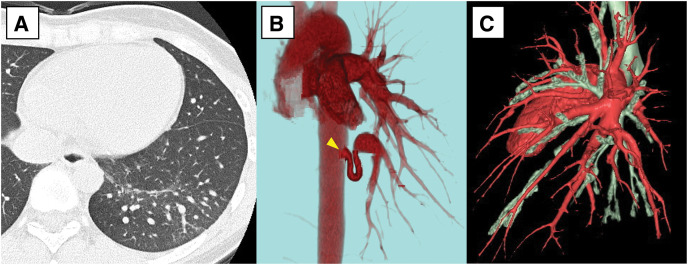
Preoperative contrast-enhanced chest CT scan of Case 2. (A) Lung window image showing extremely mild background pulmonary congestion localized to S9–10. (B, C) 3D-CT reconstructions showing an aberrant artery arising from the descending thoracic aorta (yellow arrowhead), with no pulmonary arterial defect.
